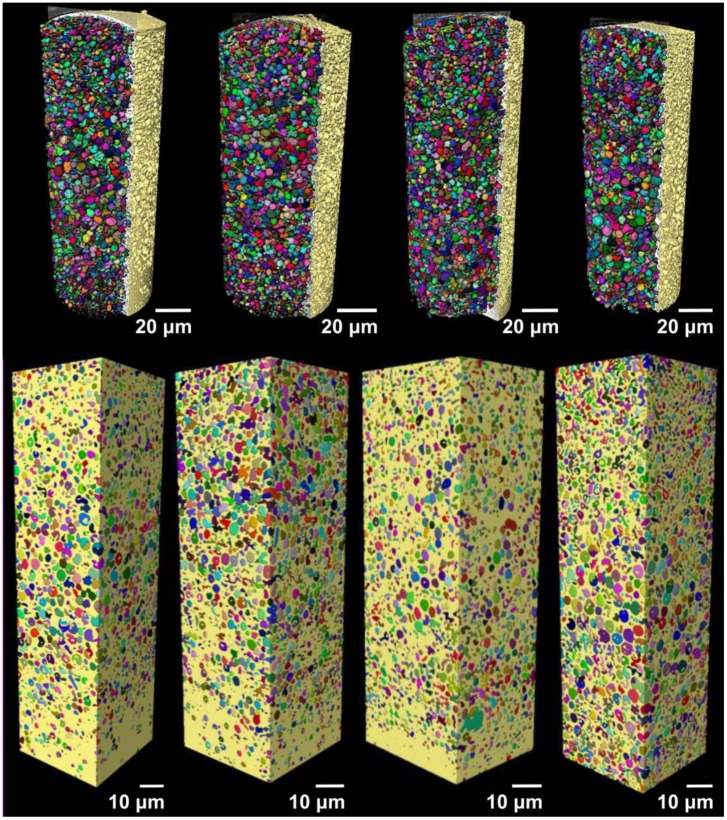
Volumetric representation of the inner part of the microspheres imaged with nano-CT. On the top from left to right, the MC-IPM, MG-IPM, MG-MCT, and MC-MCT microspheres pseudocolored in yellow are shown, with the dispersed internal spherical structures (ISS) represented in different colors. On the bottom, the volume used for the estimations done with nano-CT are shown for all samples.
